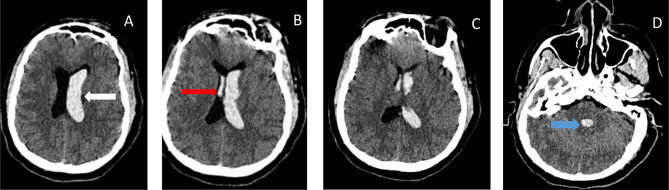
Initial non-contrast axial CT scan showing Images demonstrate extensive acute intraventricular hemorrhage (IVH). Hyperdense blood fills the left lateral ventricle (white arrow, image A), third ventricle (red arrow, Image B), and fourth ventricle (blue arrow, image D), indicating hemorrhage primarily confined to the ventricular system. There is no evidence of a large parenchymal hematoma or significant mass effect. The findings are consistent with hypertensive hemorrhage, likely secondary to the severe hypertension precipitating Posterior Reversible Encephalopathy Syndrome (PRES)
